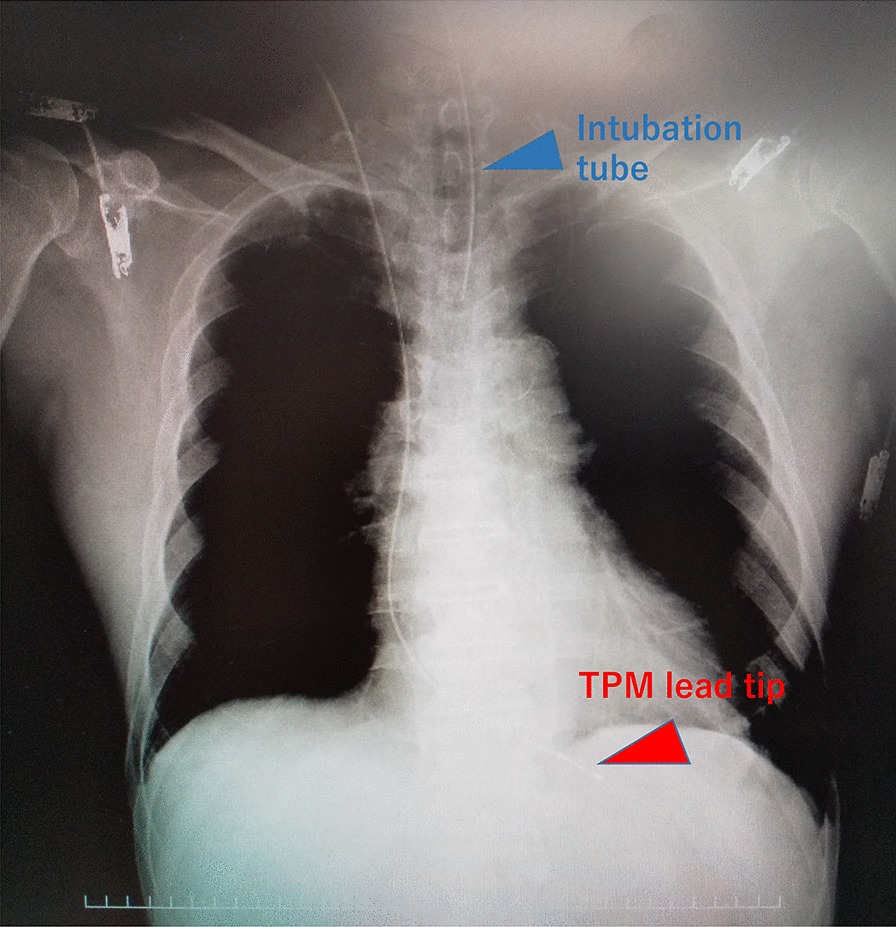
Intraoperative chest X-ray image after temporary pacemaker (TPM) insertion. There is an inserted pacemaker lead
